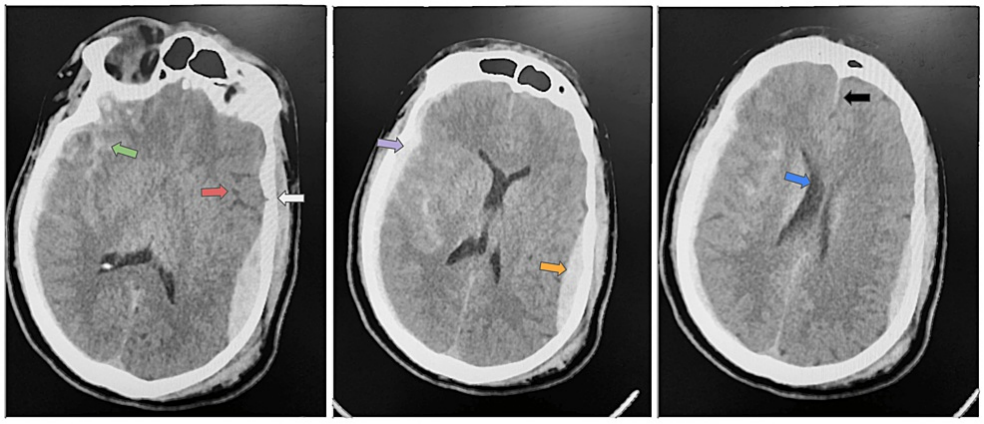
First series of head CT scans after the initial fall. Radiology report reveals a 5-mm shift of septum pellucidum from the right to left (blue arrow), areas of hemorrhagic contusion shear injury in the left temporal lobe (red arrow), and fracture at the left temporal squamosa (white arrow). Epidural hemorrhage can be seen in the left temporal region (orange arrow). Contrecoup injury can be seen in the right temporal region (green arrow). Acute subdural bleed can be seen along the right side of the temporal region (purple arrow).
